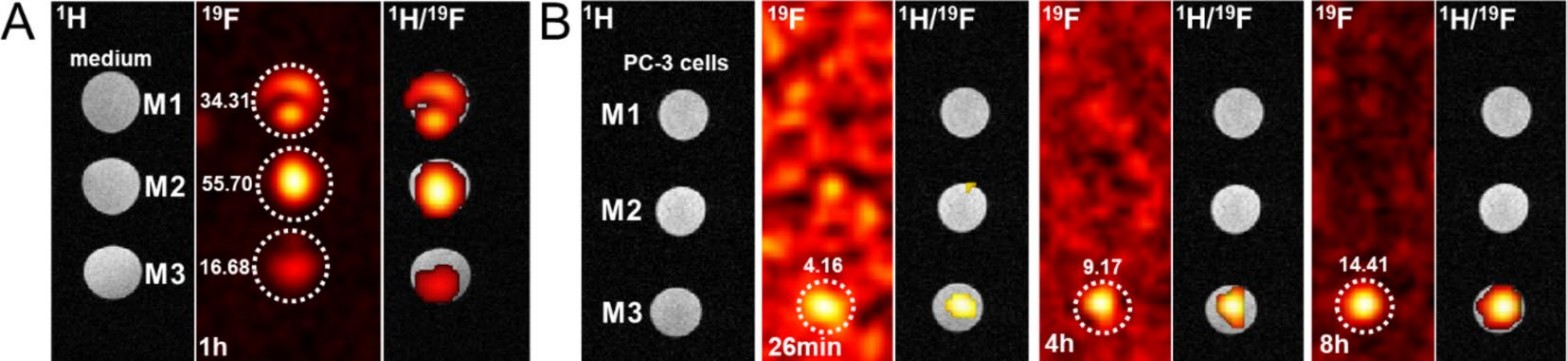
^19^F-MR results of different micelles. (A) ^1^H/^19^F-MRI/MRSI of M1 – M3 micelles in cell culture medium (*c*_*pol*_ = 20 mg mL^− 1^), scan time = 1 hour. (B) ^1^H/^19^F-MRI/MRSI of M1 – M3 micelles in labeled cells (*c*_*pol*_ = 20 mg mL^− 1^), scan time = 26 min, 4 h and 8 h. All measurements were obtained from a surface coil and simultaneously for every probe set. The overlaid ^1^H/^19^F-MRI is presented with the fluorine signal artificially marked in red.
